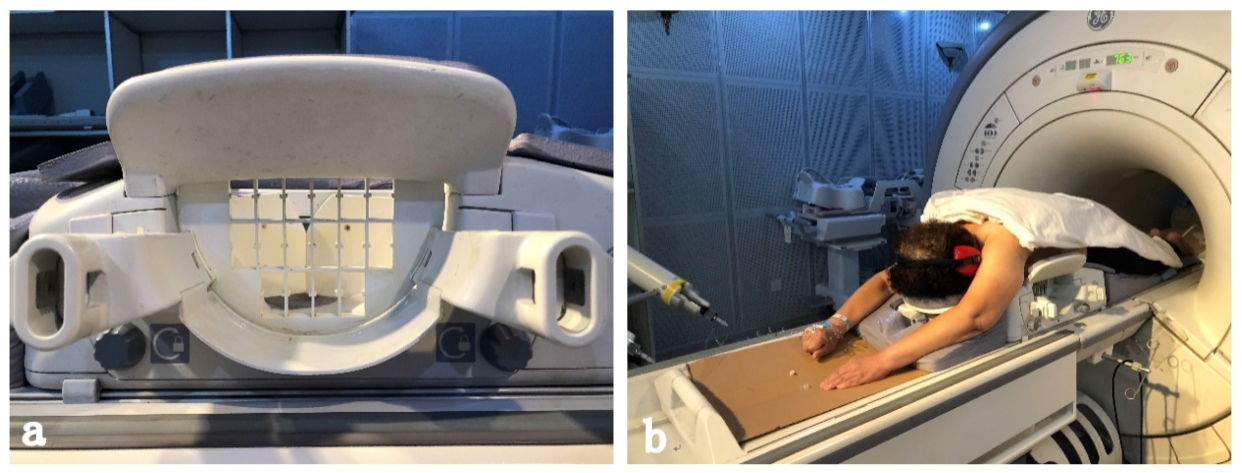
Preparations are significant for successful MRI- guided breast intervention. The coil (a)which is designed for MRI-guided intervention. A Patient is positioned prone in the coil (b) for the examination of MRI, with a mild compression for the breast. A headphone connected to the machine is used to reduce the noise of the MRI room. The arms of the patient are positioned above the head to avoid wrap artifacts and some newer coil techniques allow the arms to be positioned at the sides.
